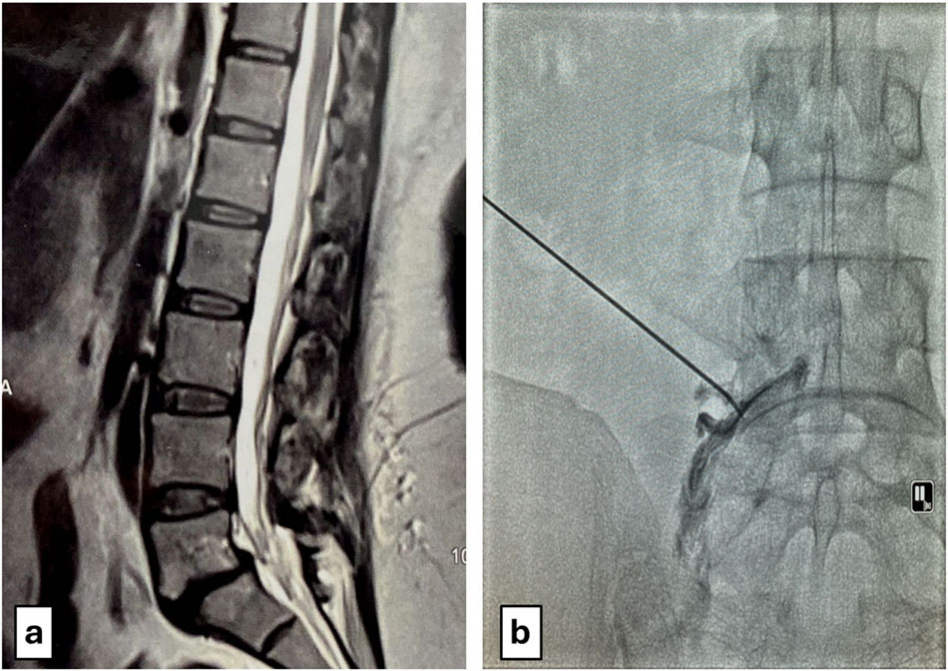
a Sagittal T2-weighted MRI of the lumbosacral spine showing diffuse posterior disc bulge at the L5‒S1 level in a 45-year-old female, with mild anterolisthesis and grade II end plate changes. Clinical examination revealed LBP with sciatica and referred pain in the L5 dermatome. b Fluoroscopic-guided image demonstrating contrast distribution along the left L5 nerve root sheath
